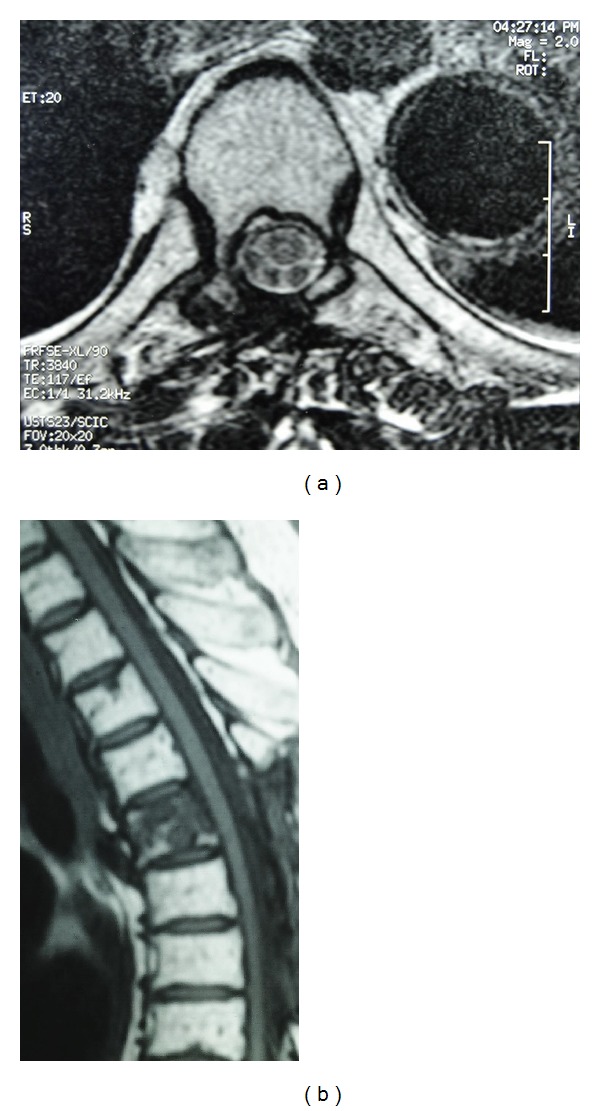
Postoperative axial (a) and sagittal (b) T1-weighted MR images showing extradural tumor removal and spinal cord decompression.
